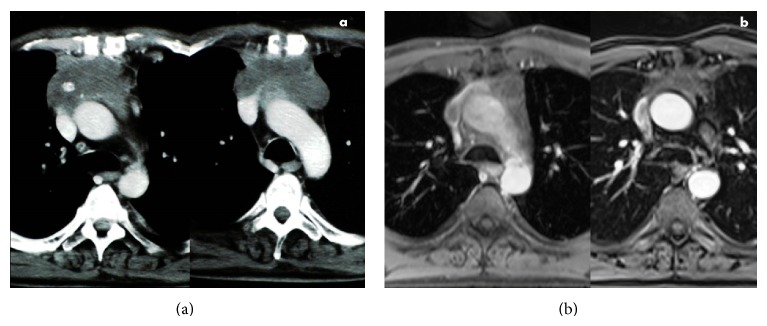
CT scans showing the local conditions of the patient one month after percutaneous RFA (a) and NMR depicting complete response 36 months after treatment (b).
